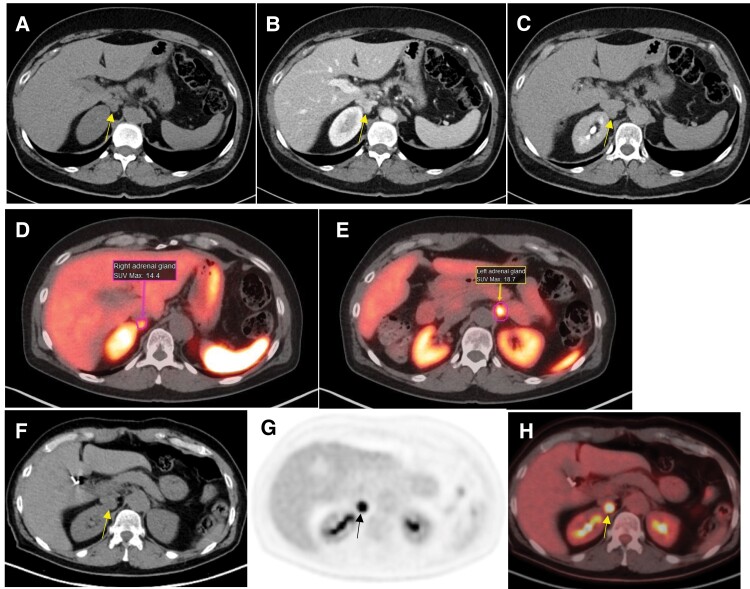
Axial computed tomography (CT) demonstrates a 12 × 9 × 8-mm right adrenal nodule. The right adrenal nodule exhibits an unenhanced CT attenuation of 31 Hounsfield units (HU) (A). The venous phase shows marked enhancement (160 HU) of the adrenal nodule (B). The nodule exhibited 15-minute delayed attenuation of 68 HU; the calculated 71% absolute washout was consistent with a benign lesion (C). ^68^Ga-DOTATATE PET/CT was nondiagnostic for pheochromocytoma, showing a maximum standardized uptake value (SUVmax) of the right adrenal gland of 14.4, less than that of the left adrenal gland SUVmax 18.7 (D and E); this was thus interpreted as physiologic distribution of radiotracer within bilateral adrenal glands. ^18^F-FDG PET/CT demonstrated the right adrenal nodule (F), with ^18^F-FDG axial stand-alone PET image showing intense right adrenal uptake (G). The fused ^18^F-FDG PET/CT image confirmed the right adrenal nodule was highly avid, with SUVmax 23.2 (H), compatible with a right adrenal pheochromocytoma.
